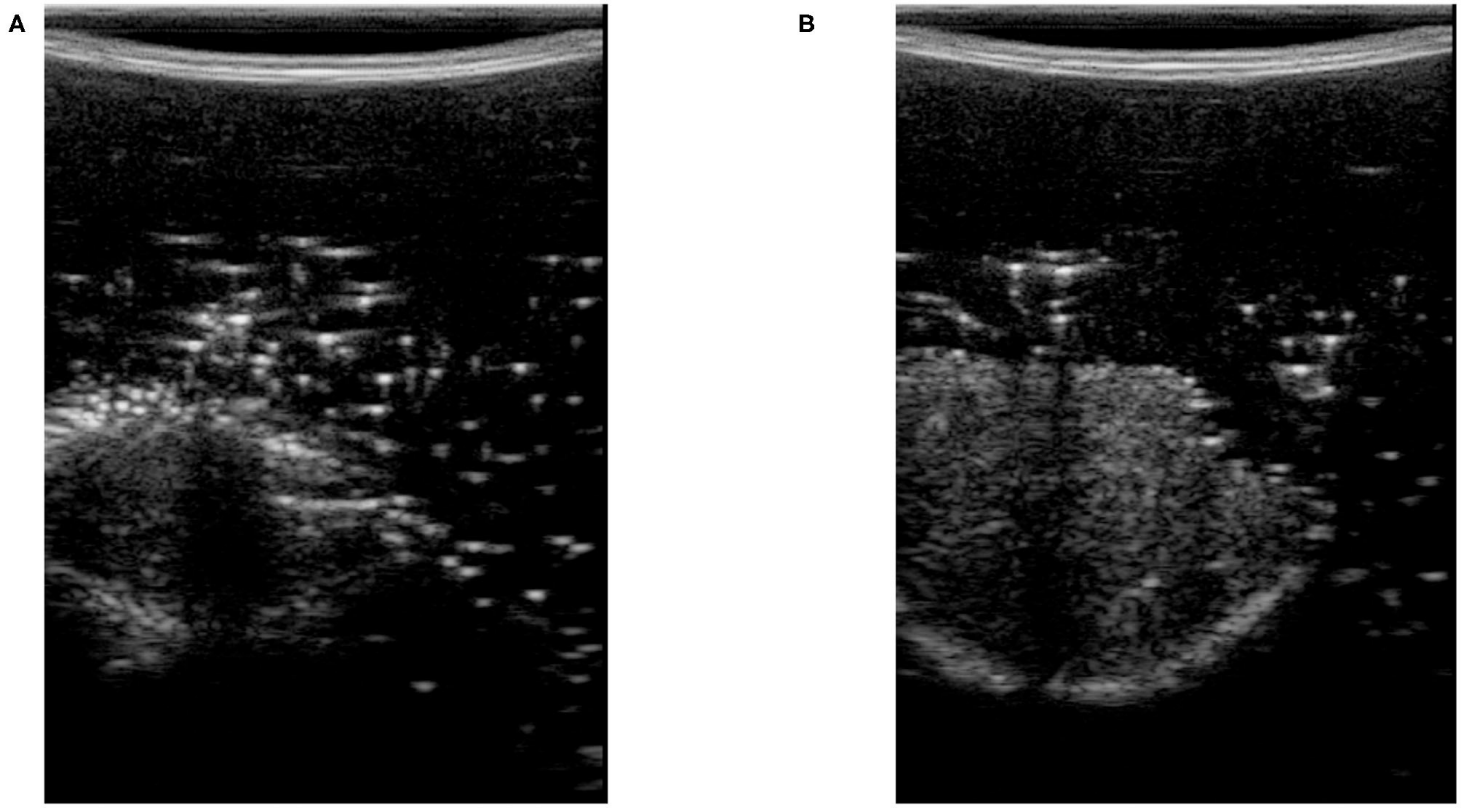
Ultrasound images with high and low level of compression. (A) Ultrasound image with high tissue compression. (B) Ultrasound image with low tissue compression.
